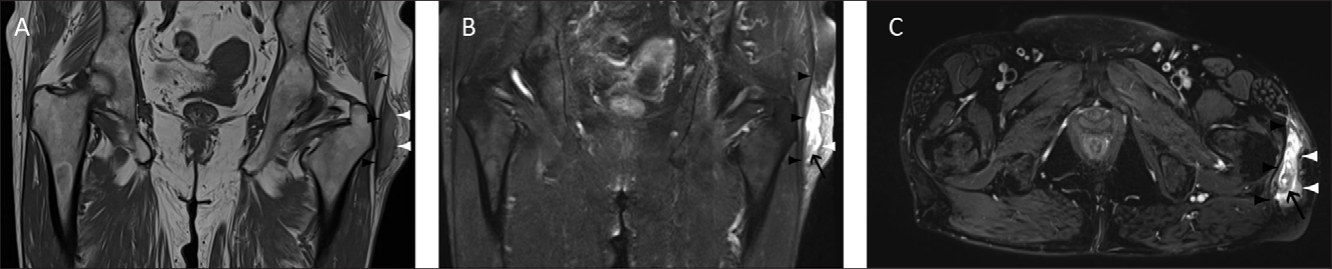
A 58-year-old man presenting with a left hip mass. Type III Morel-Lavallée lesion: chronic organizing hematoma. Coronal T1 (A), coronal PD FS (B), and axial PD FS (C) MR images of the left hip show a complex mass between the fascia lata (white arrowheads) and the iliotibial tract (black arrowheads). The mass has an intermediate SI on T1-WI and high SI on T2-WI. The inhomogeneity of the collection represents the separation of blood products and debris (black arrow). A peripheral, thickened, low SI capsule is seen.
